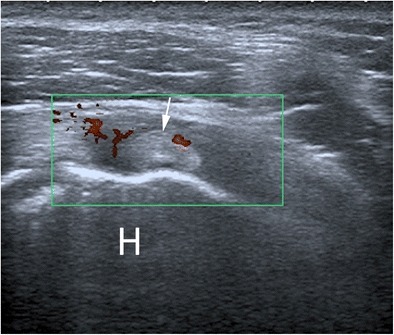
Evaluation of the rotator interval in a patient with adhesive capsulitis for 3 weeks. The power Doppler signal is clearly seen within hypoechoic scar tissue (*asterisks*). *H* humerus, *arrow* biceps tendon
